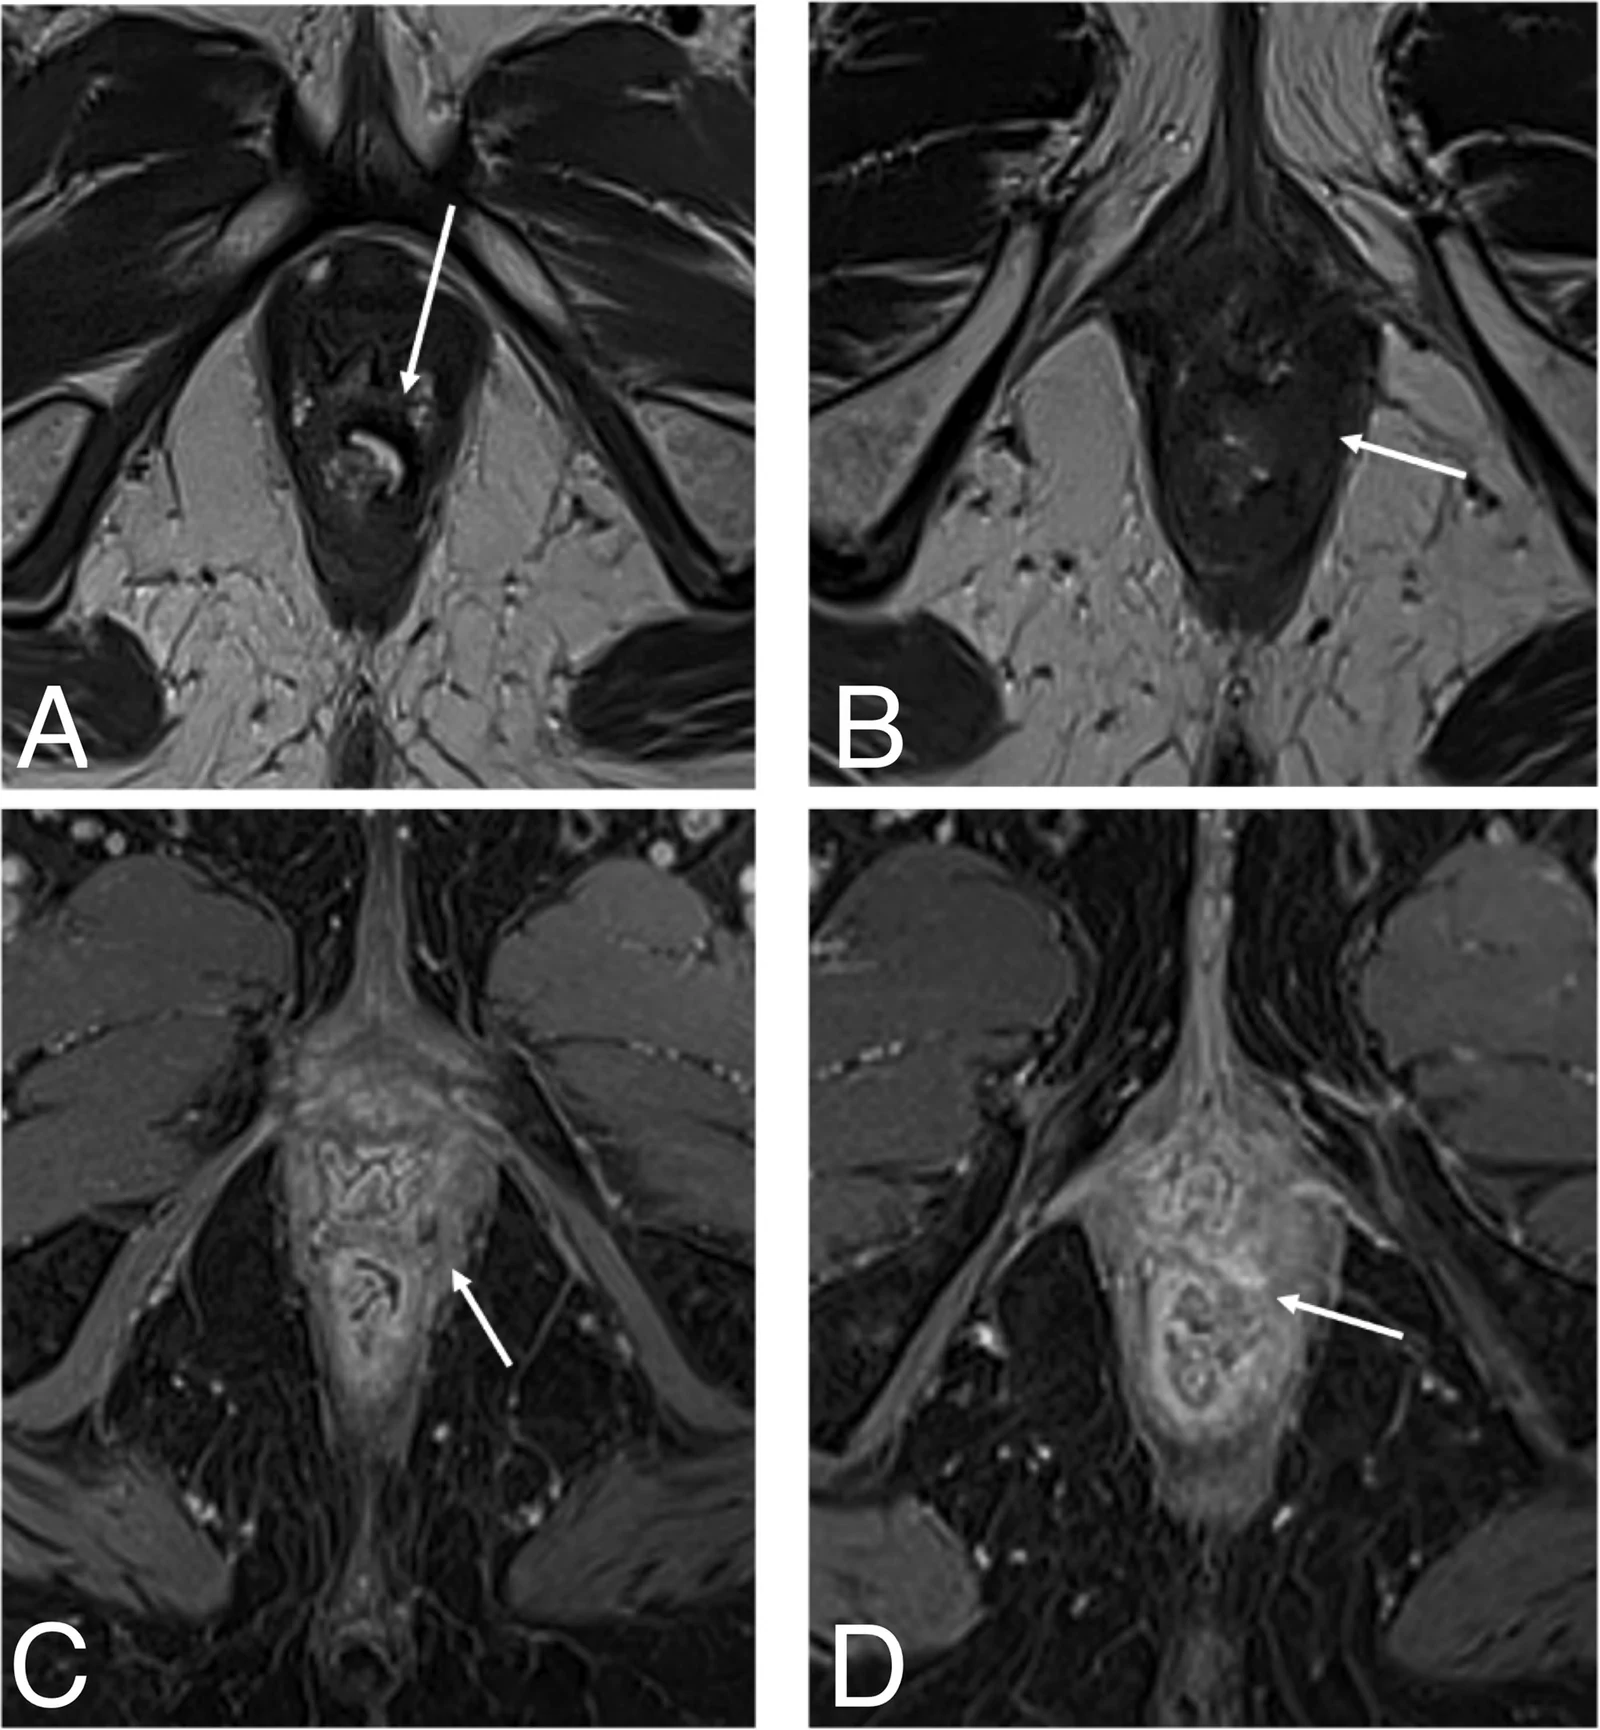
Female anal canal cancer patient initially managed with chemoradiation therapy. Follow up MRI at 9 months after chemoradiation therapy shows a complete response on axial oblique high resolution T2-weighted image (A, arrow) and post contrast axial oblique T1-weighted image (C, arrow). 18 months later, axial oblique high resolution T2-weighted image (B) and post contrast axial oblique T1-weighted image (D) demonstrate anterior local recurrence abutting the left puborectalis muscle and infiltrating the posterior vaginal wall (arrow). Key point: evaluation in true axial and axial oblique planes helps distinguish subtle regrowth from treated tissue, especially anteriorly.
